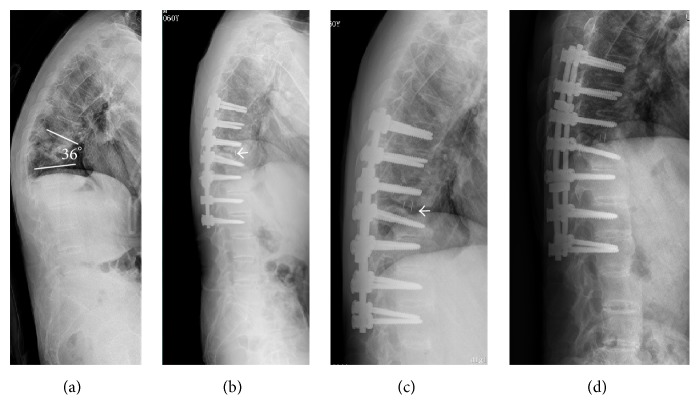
A 59-year-old male patient presented with severe back pain and progressive kyphotic deformity. Lateral radiograph (a) before surgery showed local kyphosis of 36° and pseudarthrosis at T10-T11 level. Immediately after surgery lateral X-ray (b) revealed transpseudarthrosis osteotomy and interbody fusion with PEEK cage (arrow). Three months after surgery, normal sagittal alignment was maintained and partial union (c, arrow) was obtained. At final follow-up, no evidence of nonunion and no loss of correction on lateral X-ray (d).
